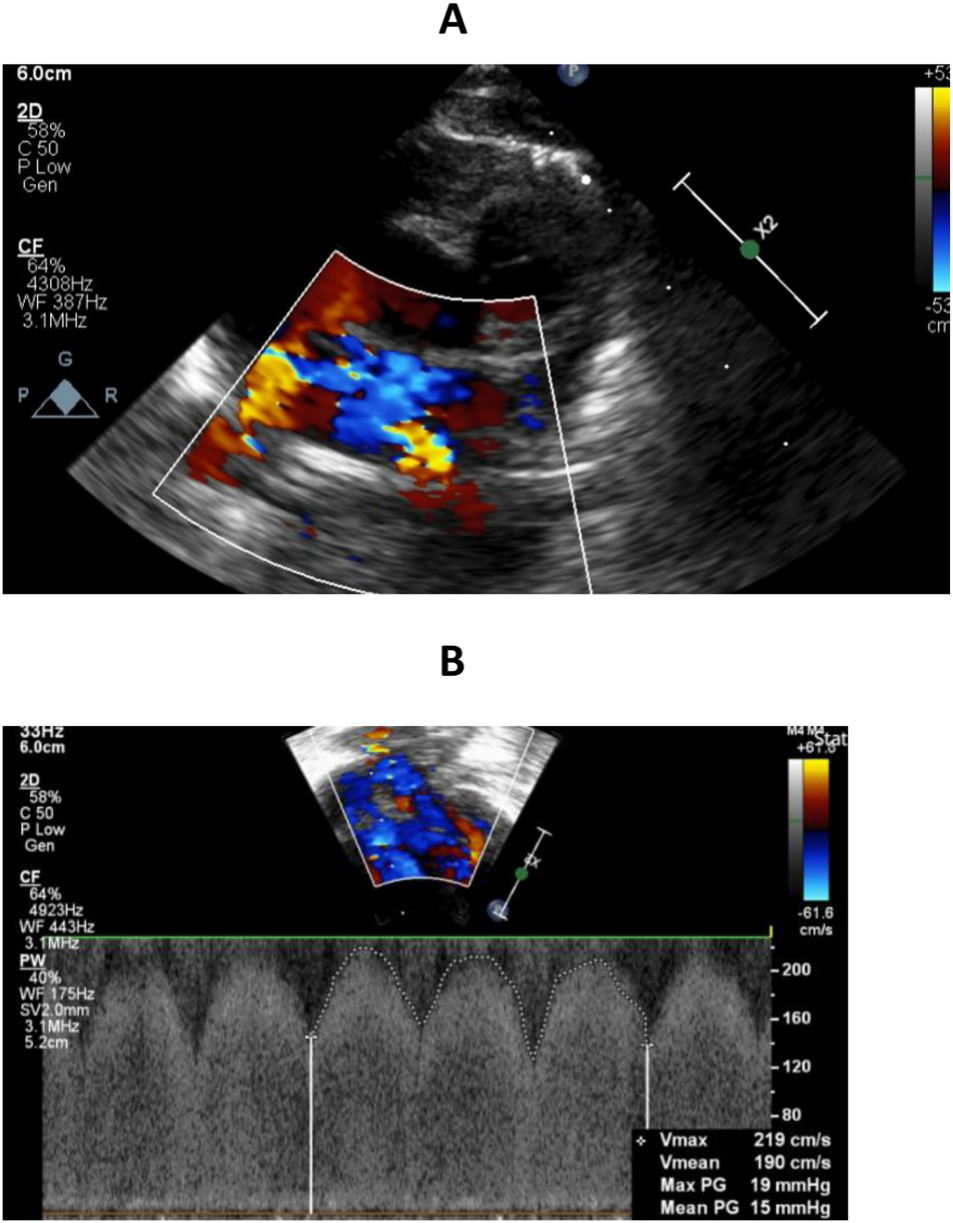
Pre dilation echo. (A) 2D echocardiography and colour Doppler of pulmonary veins demonstrating flow turbulence in lower pulmonary veins indicative of possible stenosis. (B) 2D echocardiography and pulse wave Doppler of pulmonary vein demonstrating increased velocity (2 m/s) and mean pressure gradient (15 mmHg). Note that the pulse waveform does not return to baseline, in keeping with features of an obstructed pulmonary vein.
